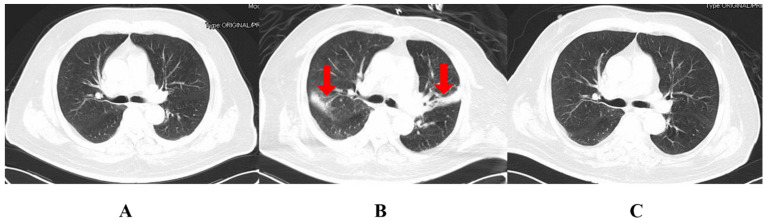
The CT of the chest during the patient’s hospital stay. (A): the Chest CT on 8 February (two days before surgery); (B): the Chest CT on 4 March (day 22); (C): the Chest CT on 22 April (day 71). The areas indicated by the red arrows are the sites of infection.
